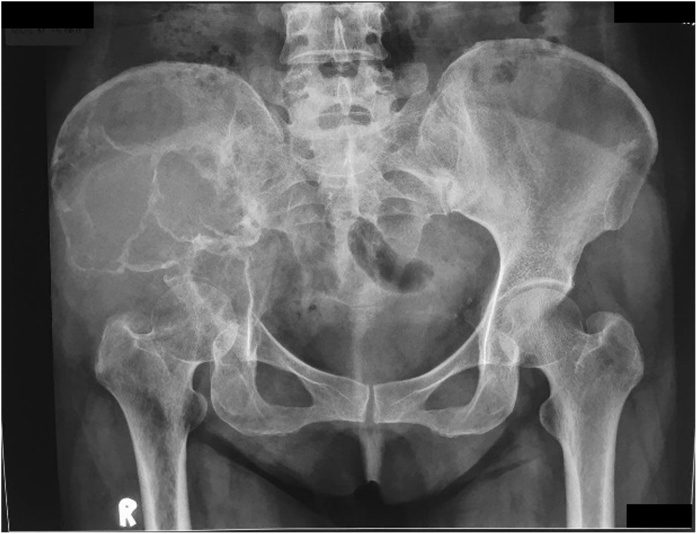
Pelvic x-ray showing destructive lytic lesion involving right ilium and ischium.
